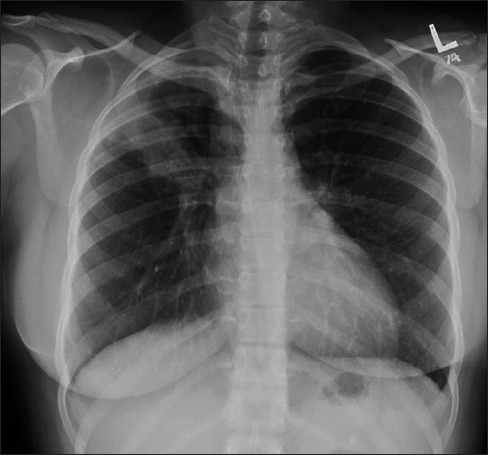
A frontal radiograph shows a segmental collapse of the right upper lobe. Note the elevation of the lesser fissure and the right hilum and a minor mediastinal shift to the right. This was an asthmatic patient, with a mucus plug
